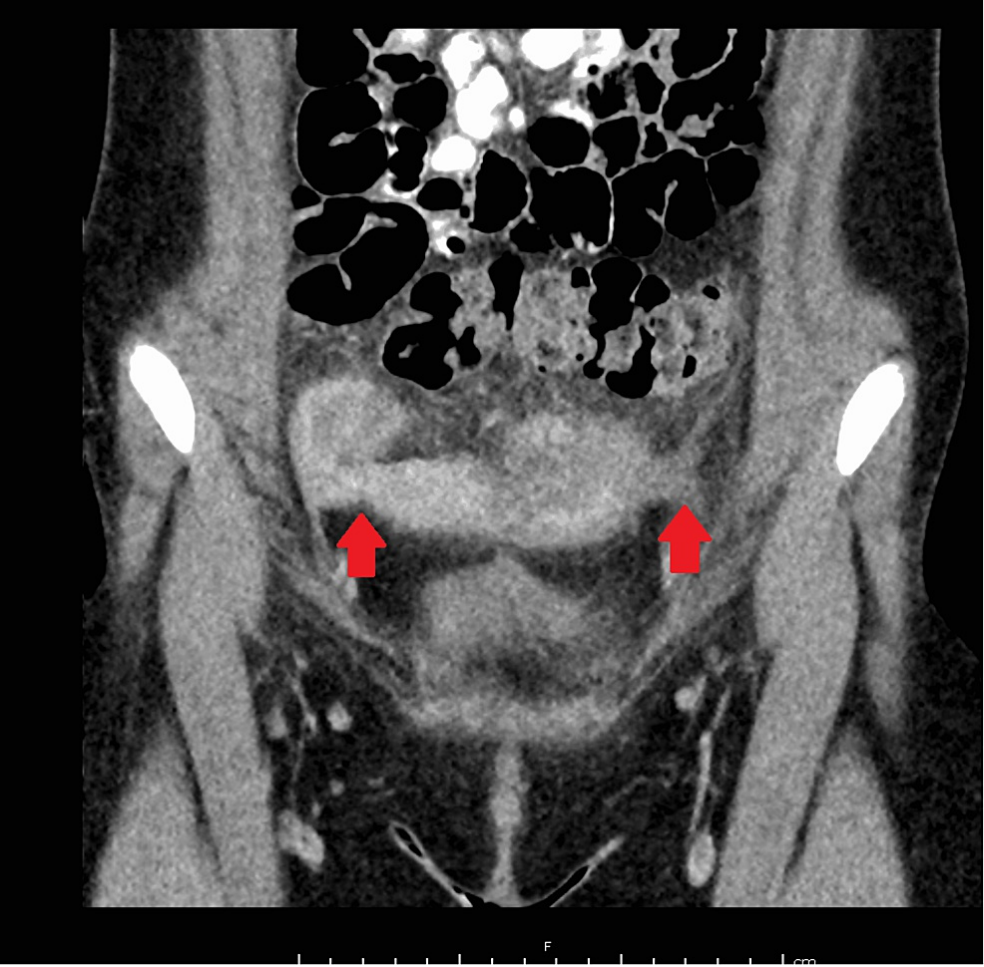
CT abdomen showing bilateral hydrosalpinx right greater than left
